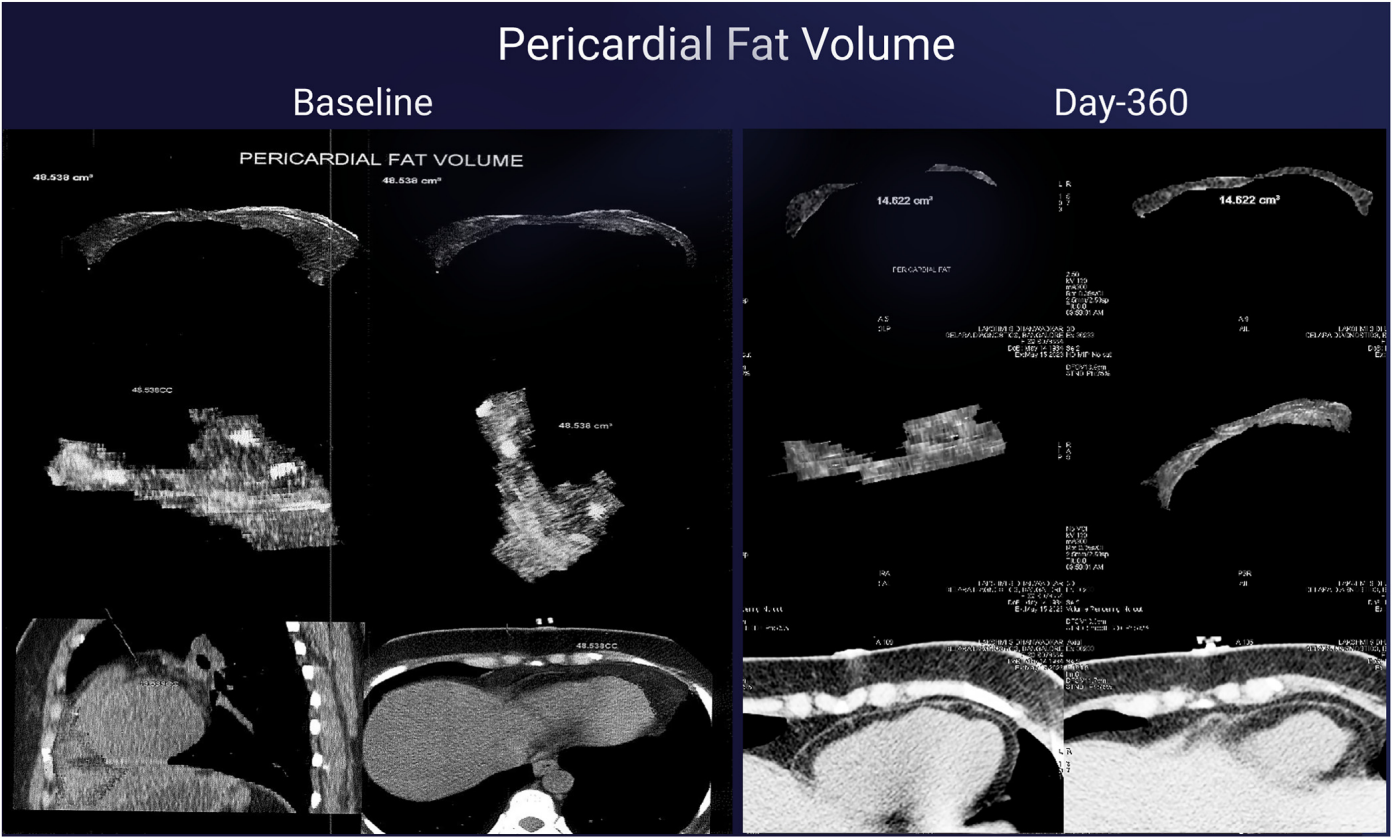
Pericardial fat volume measured by computed tomography (CT) scan at baseline and day 360 pericardial volume from 48.54 cm3 at baseline to 14.6 cm3 at day-360 (69.9% reduction).
